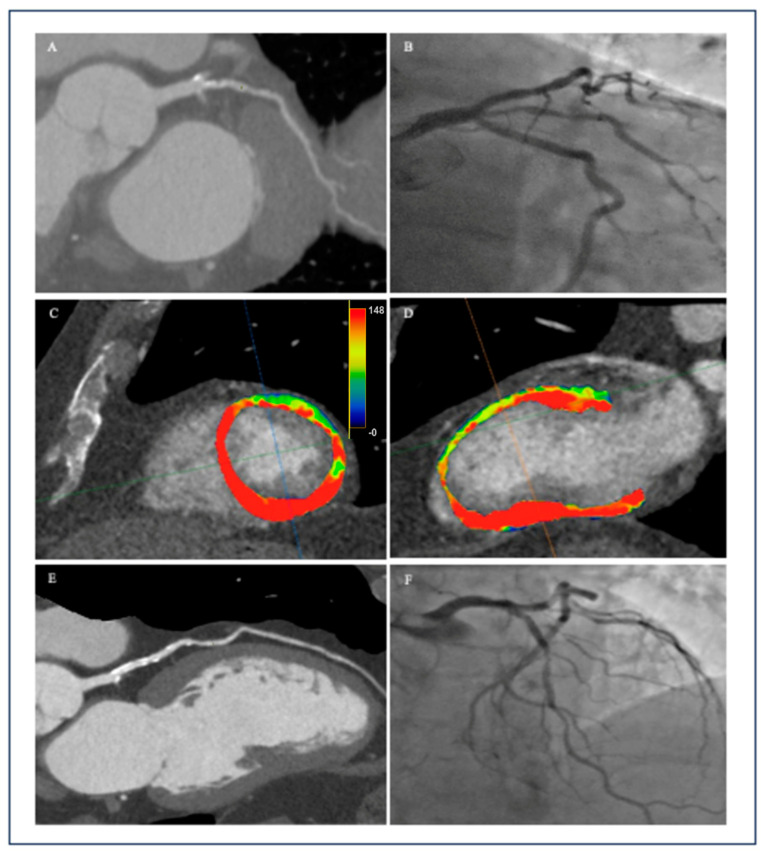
A 68-year-old male with atypical angina and the following cardiovascular risk factors; hypertension, diabetes type 2, hyperlipidemia, nicotine dependence, obesity (BMI 33 kg/m^2^). The patient underwent CT coronary angiography, dynamic CT myocardial perfusion, and invasive coronary angiography. Coronary CT angiography revealed: 65% luminal narrowing [CAD-RADS: 3 in the ramus intermedius artery (A); 65% luminal narrowing [CAD-RADS: 3/N] in the left anterior descending artery (E). Invasive coronary angiography revealed: 75% stenosis in the proximal ramus intermedius artery (B); 30% stenosis in proximal LAD, 70% stenosis in mid-LAD, and severe stenosis in diagonal branches (F). Regadenoson-stress myocardial CT perfusion with dynamic acquisition: ((C)—short axis and (D)—2-chamber view) revealed perfusion defect with decreased MBF in the mid anterior, mid-anterolateral, and apical anterior segment. Scan protocol included: 400 mg of regadenoson and 45 mL of nonionic iodinated contrast. FFR measurements performed during ICA-FFR value of 0.75 indicating significant changes in LAD and FFR value of 0.78 indicating significant changes in the intermediate branch.
